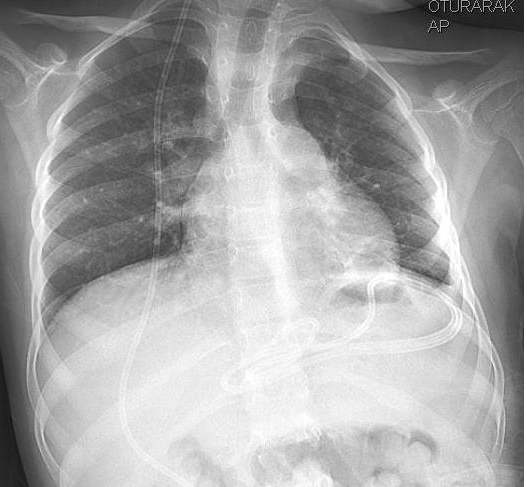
In chest roentgenogram, the ventriculoperitoneal shunt located in the left subdiaphragmatic region.
